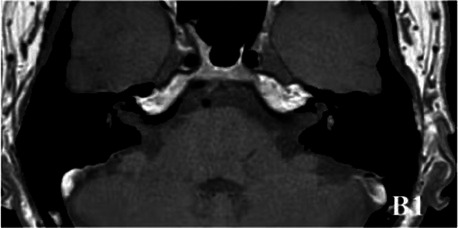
In October 2019, the signal of the left vestibular in the T1WI sequence remained higher than that in the contralateral (B1). In the 3D-T2-FLAIR sequence, the signals of the left vestibular and vestibular nerve were higher than those of the right (B2).
